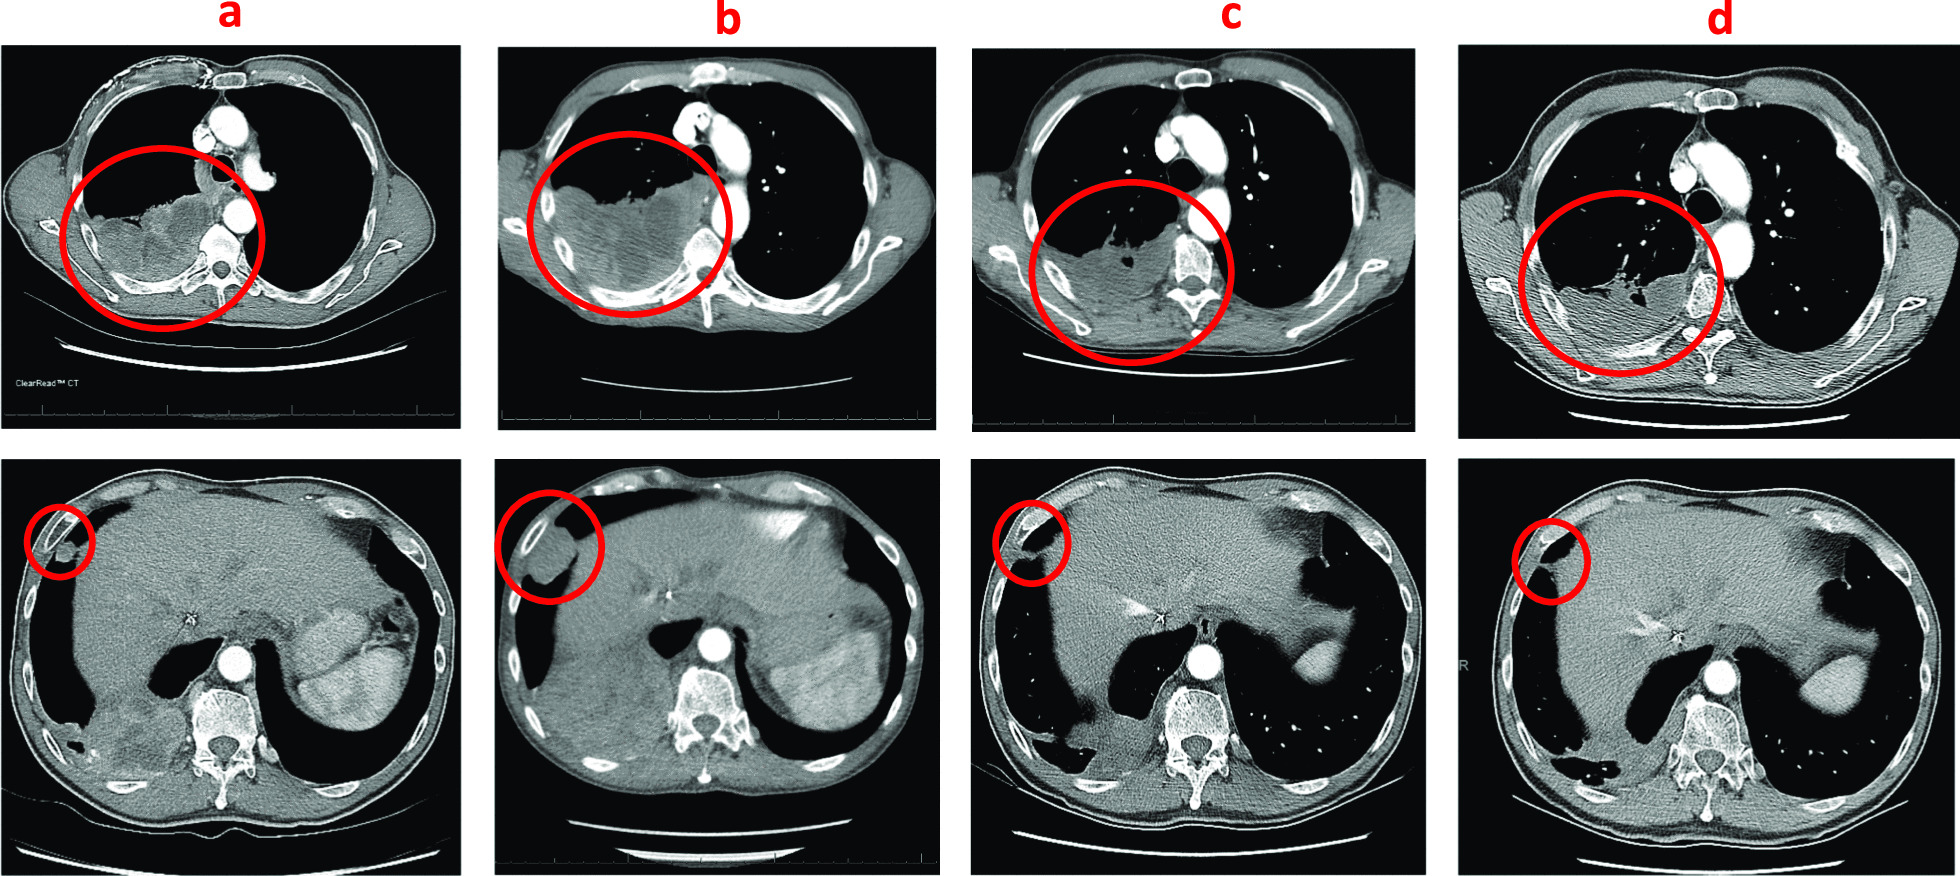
Serial computed tomography axial images. a Baseline, b after 2 cycles of chemotherapy + immune checkpoint inhibitor (ICI), c after 4 cycles of chemotherapy + ICI and d 4 cycles of maintenance ICI. Circled regions denote right-sided thoracic tumors
